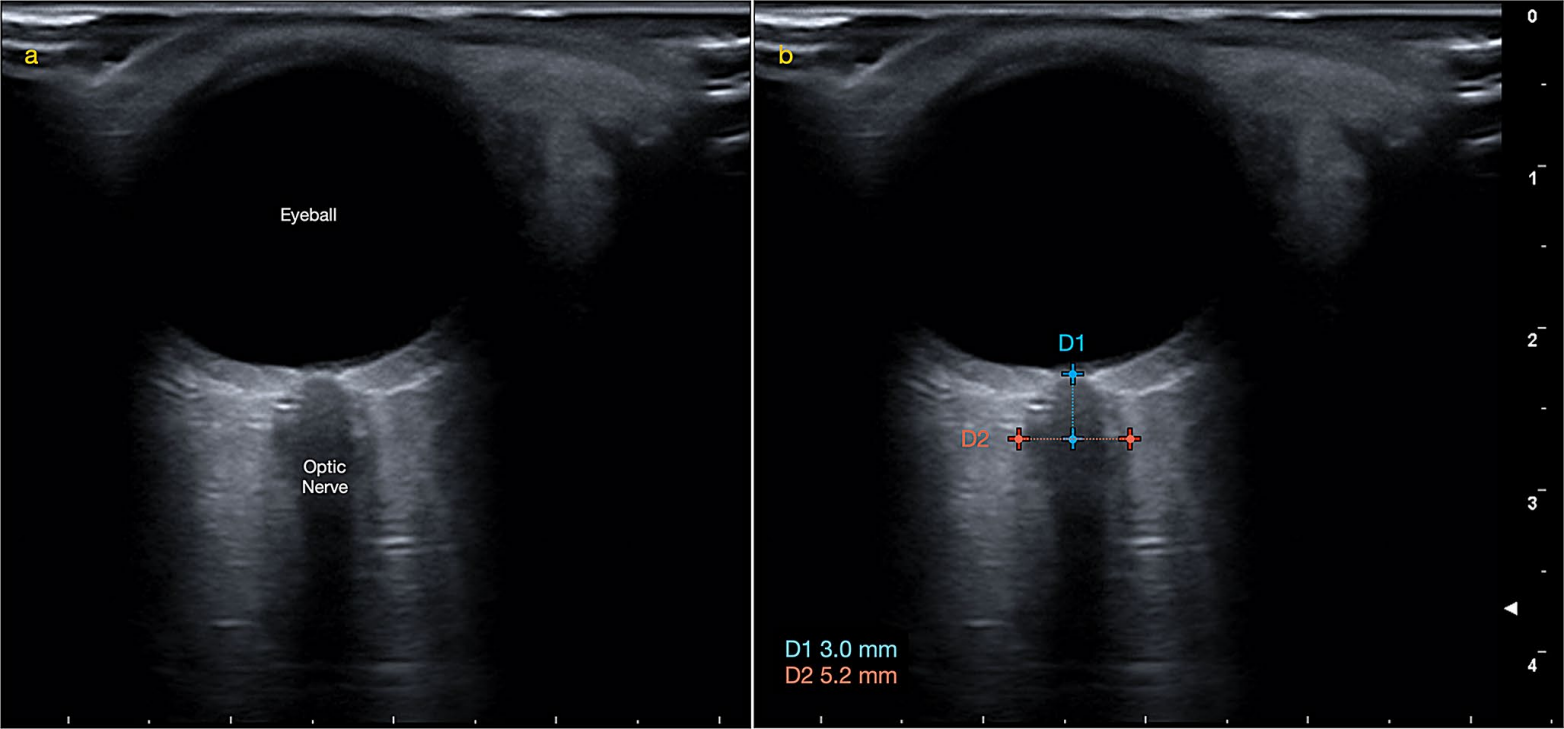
(a) The ultrasonographic image of the eyeball and optic nerve. (b) The measurement of the optic nerve sheath diameter (ONSD) was taken 3 mm behind the optic disc
